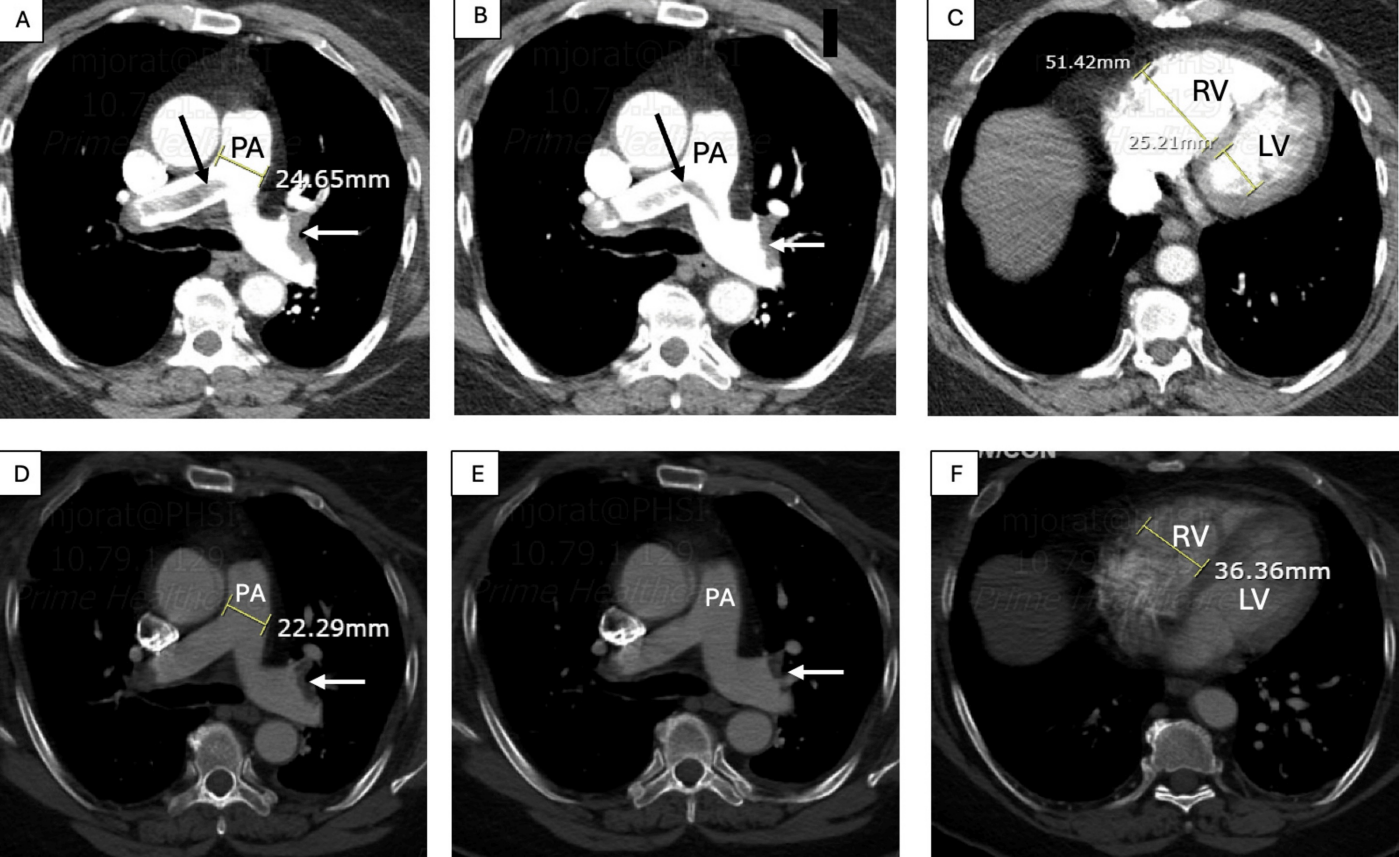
Chest CTA before and after receiving tenecteplase A, B, and C: CTA before thrombolysis: saddle pulmonary emboli with extension to bilateral pulmonary arteries (black arrow) and subsegmental branches with pulmonary artery (PA) and right ventricle (RV) dilatation; PA diameter: 24.65 mm, RV diameter: 51.42 mm. D, E, and F: CTA three days after thrombolysis: significant reduction in thrombus burden with residual thrombus in distal pulmonary arteries and lower lobe branches (white arrow) and a significant decrease in PA and RV dimensions; PA diameter: 22.29 mm, RV diameter 36.36 mm, which indicate successful thrombolysis CTA: computed tomography angiography
